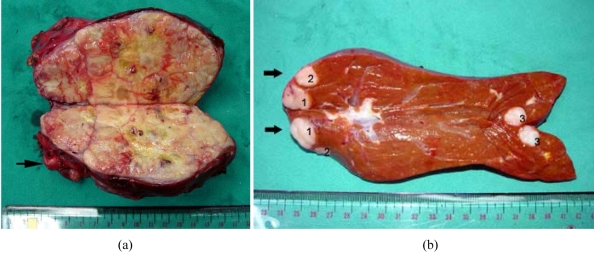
(a) Longitudinal cut section of the right adrenal gland shows solid white lobulated tissue and areas of yellow necrosis and brown haemorrhage with matted lymph nodes (arrow), which correlated to the CT findings; (b) Cut section of the right posterior segment hepatectomy shows 3 solid white nodules (1-3) with small hemorrhagic spots (arrows indicate the dome of the liver).
